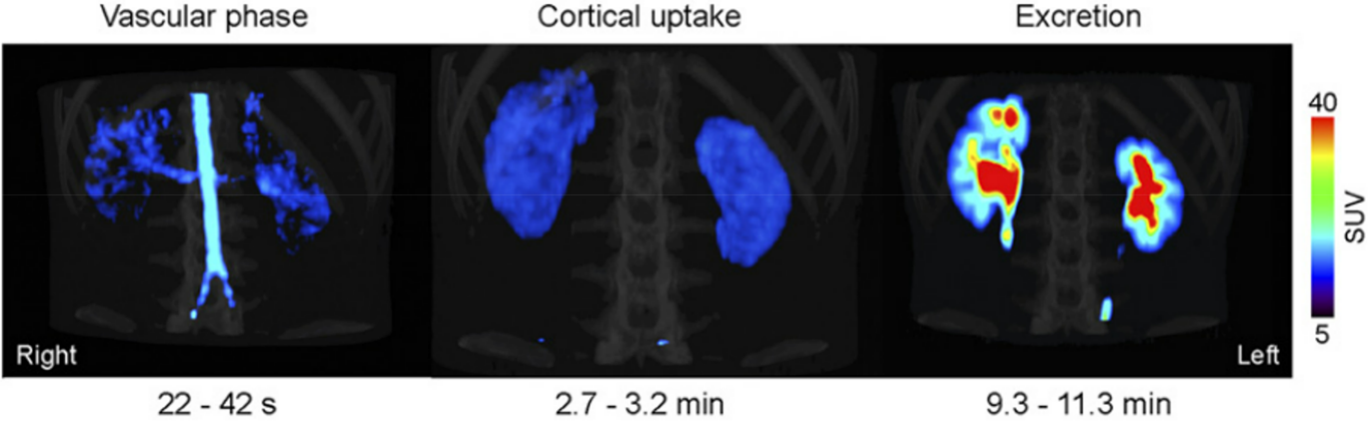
[^11^C]PABA PET/CT renal imaging in a healthy human subject.
